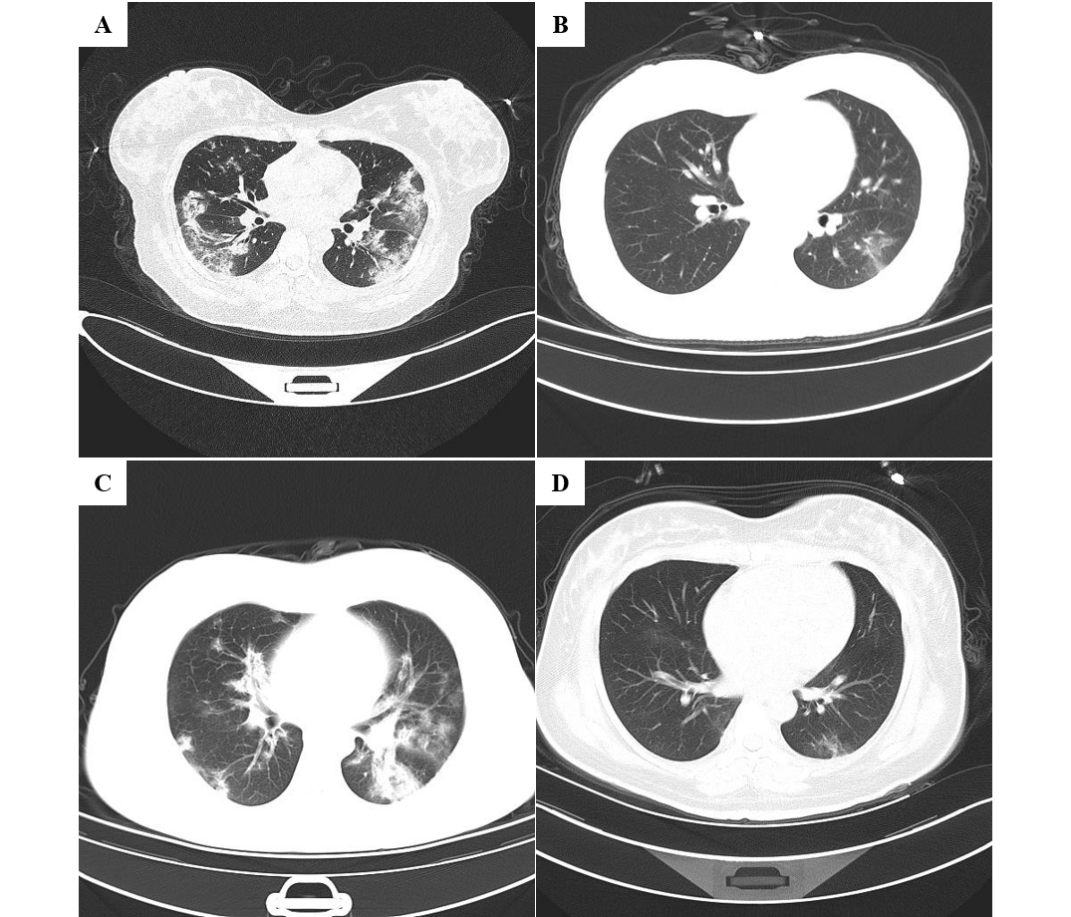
Chest computed tomography scans of 4 patients with the coronavirus disease.
